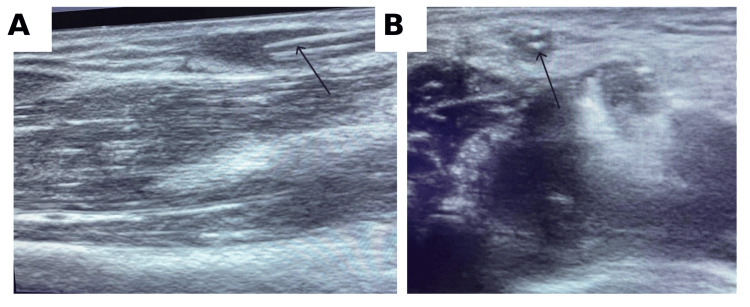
Ultrasonographic localization of the retained catheter fragment (A) Long-axis view showing a linear echogenic intraluminal structure (arrow). (B) Short-axis view confirming the retained fragment within the superficial antecubital vein (arrow).
